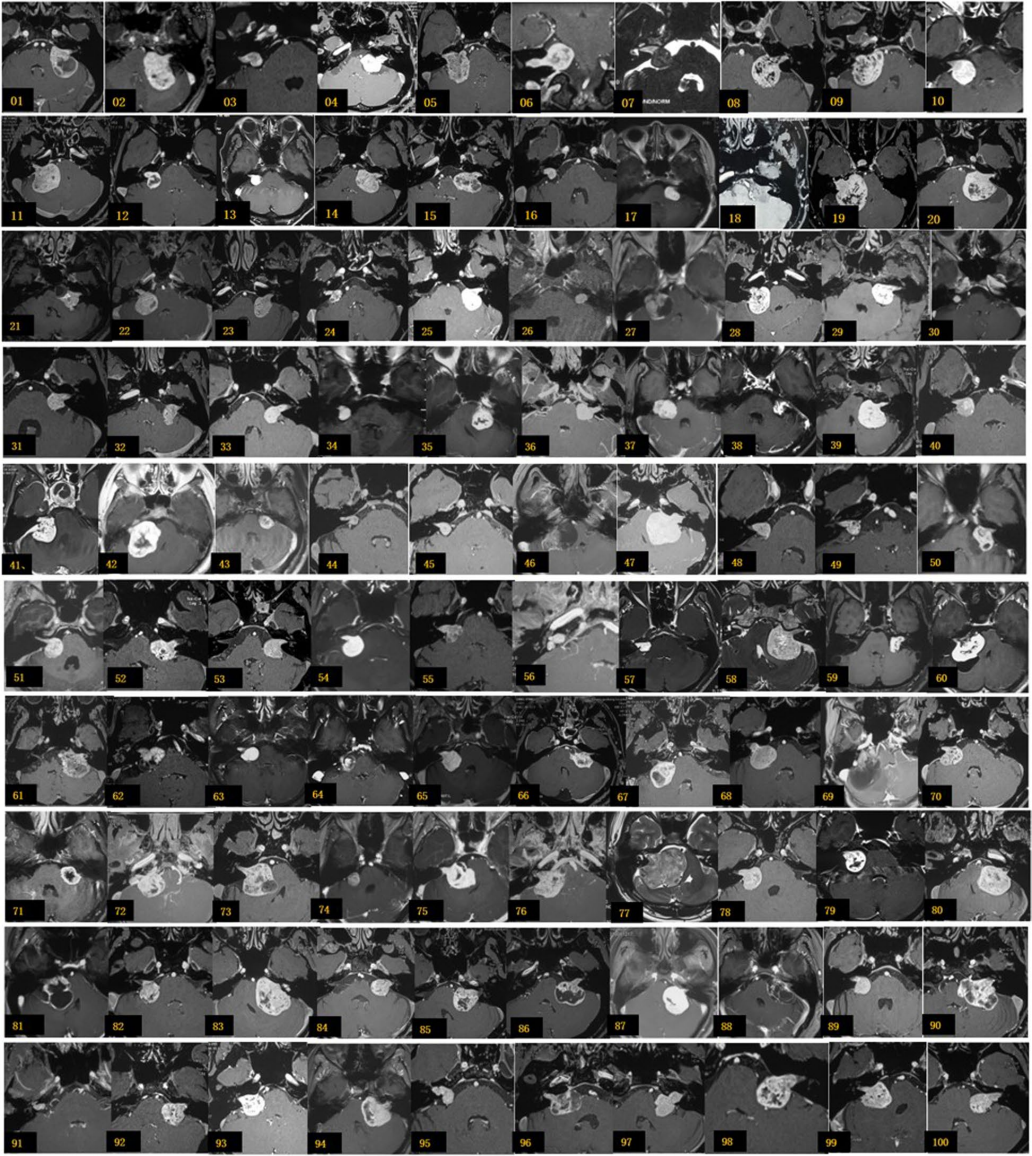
Preoperative brain MRI of the 100 cases
